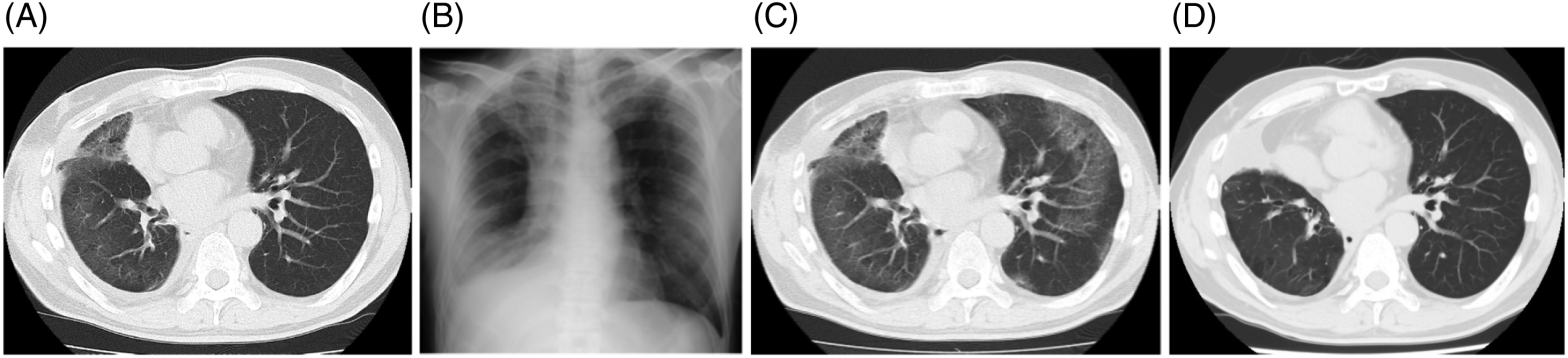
Images of chest x‐ray and computed tomography (CT). (A): day 7, (B) and (C): day 0, and (D): 9 months after the onset.
